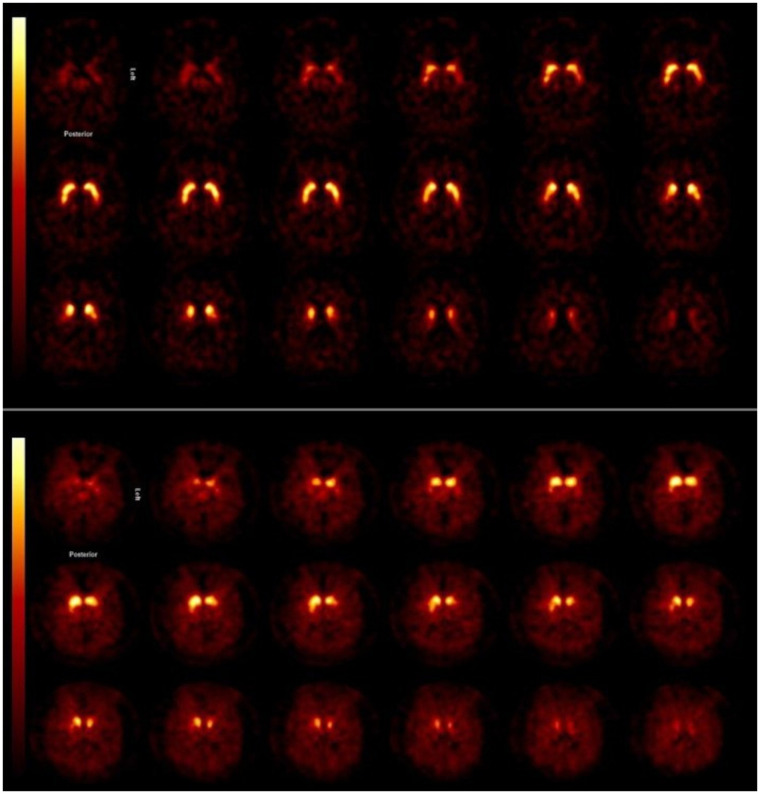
Transversal ^123^I-FP-CIT SPECT images obtained in patient with CUPS without striatal DAT loss (top) and in CUPS patient with striatal DAT loss (bottom). Asymmetric striatal binding can be seen, as well as severe loss of DAT binding, especially in putamen, in subject with dopaminergic deficit. This study was acquired on brain-dedicated SPECT system (InSPira; NeuroLogica).
